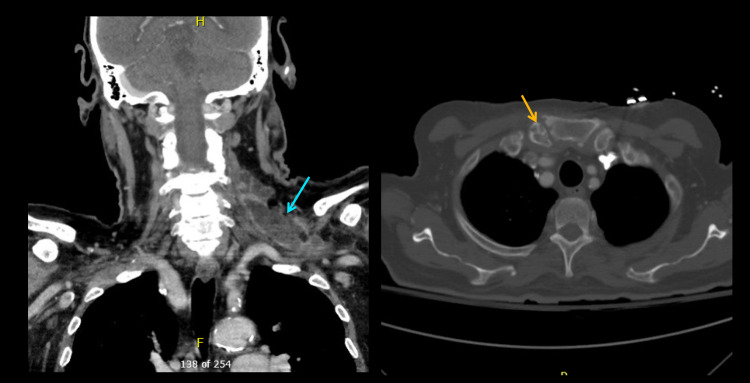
CT neck and chest Blue arrow points to growing left sternocleidomastoid abscess. Orange arrow points to right clavicle osseous erosion.
